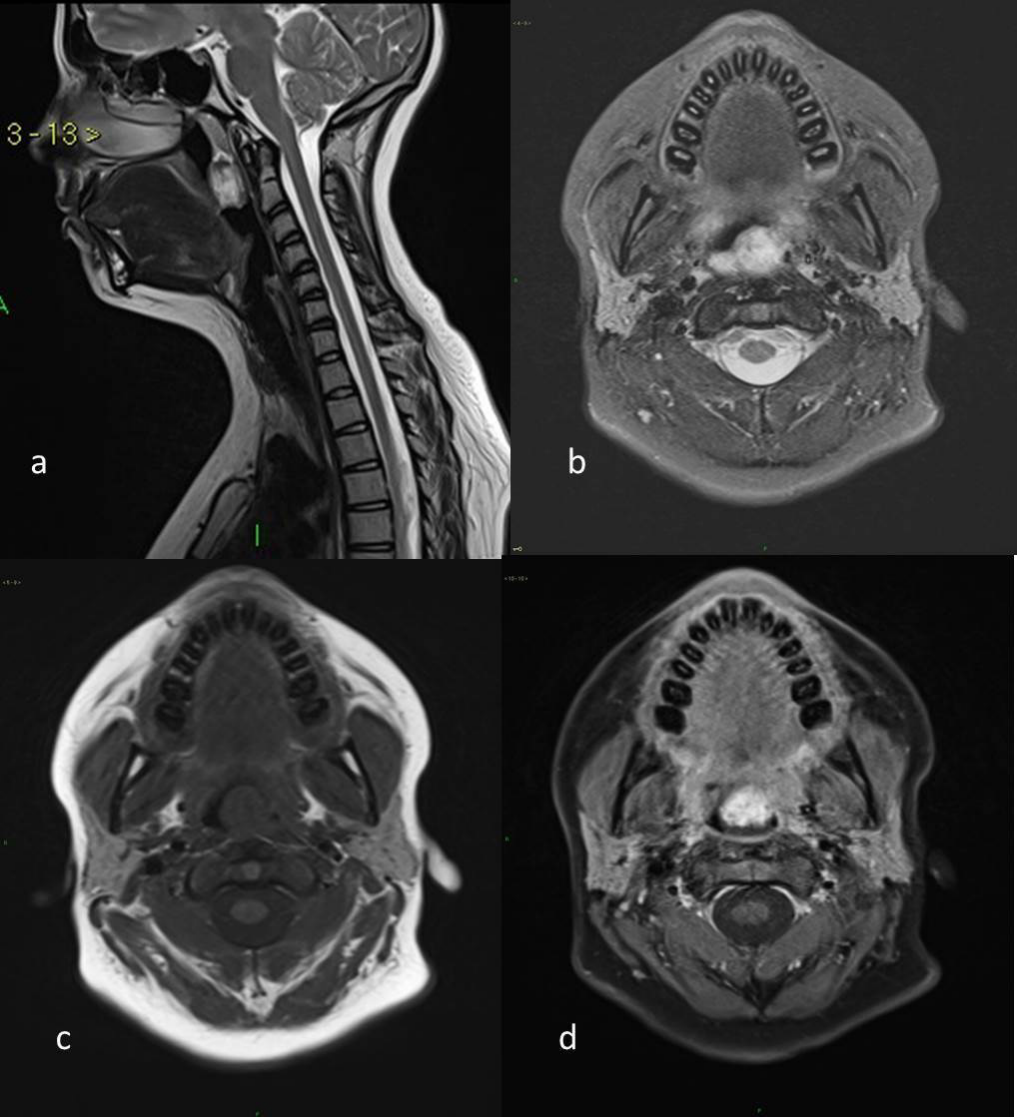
Sagittal T2 (a) and axial T2FS (b) MR images show heterogeneous hyperintense lesion in nasopharynx causing mild airway narrowing. The lesion appears isointense in ﻿﻿T1 WI (c) and enhances in post contrast T1 weighted FS sequence (d). FS, fat suppressed; T1 WI, T1 weighted imaging.
